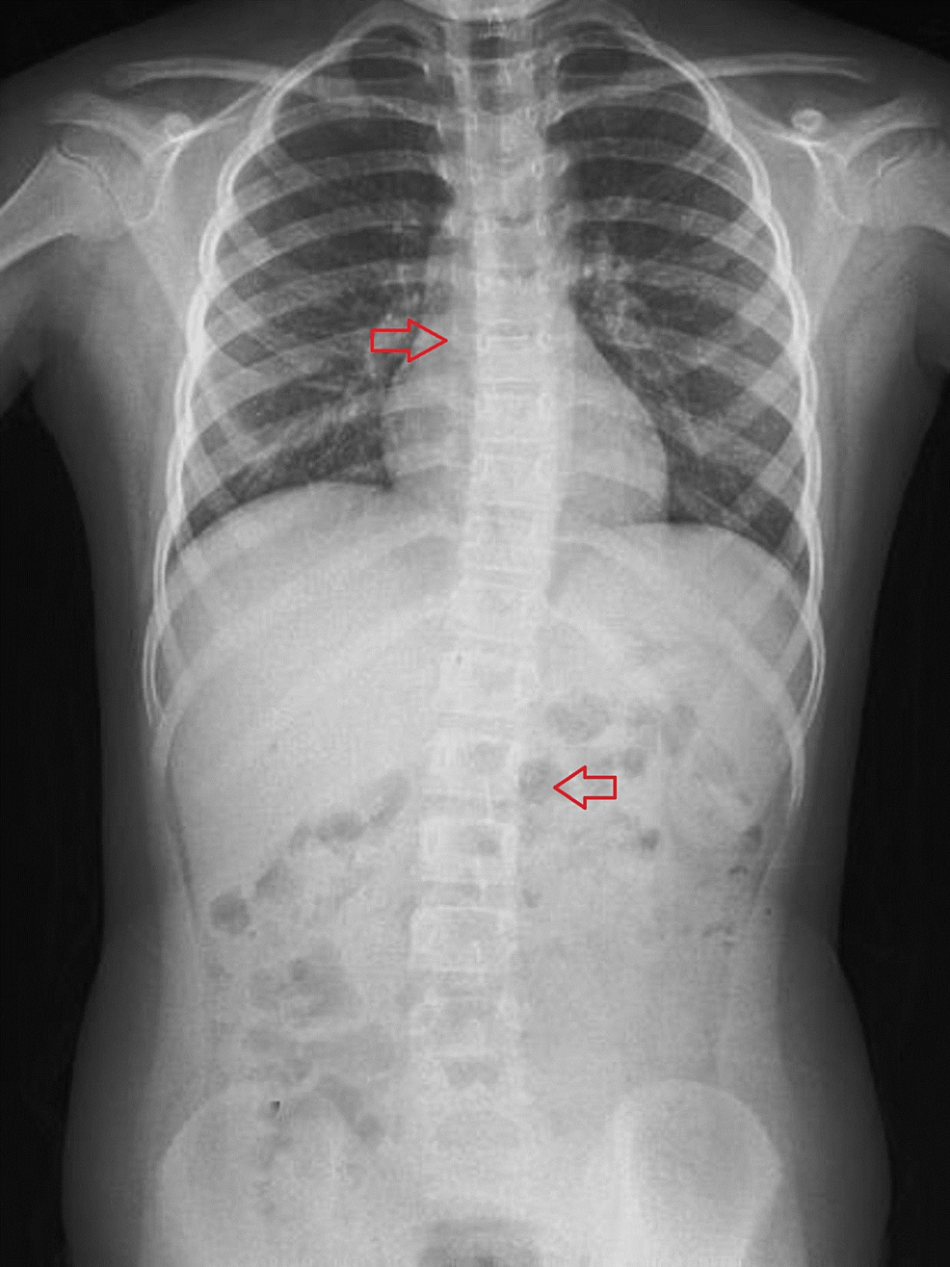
Roentgenography of an early-onset scoliosis (EOS) patient. Left and right arrows indicate the scoliotic vertebrae.
